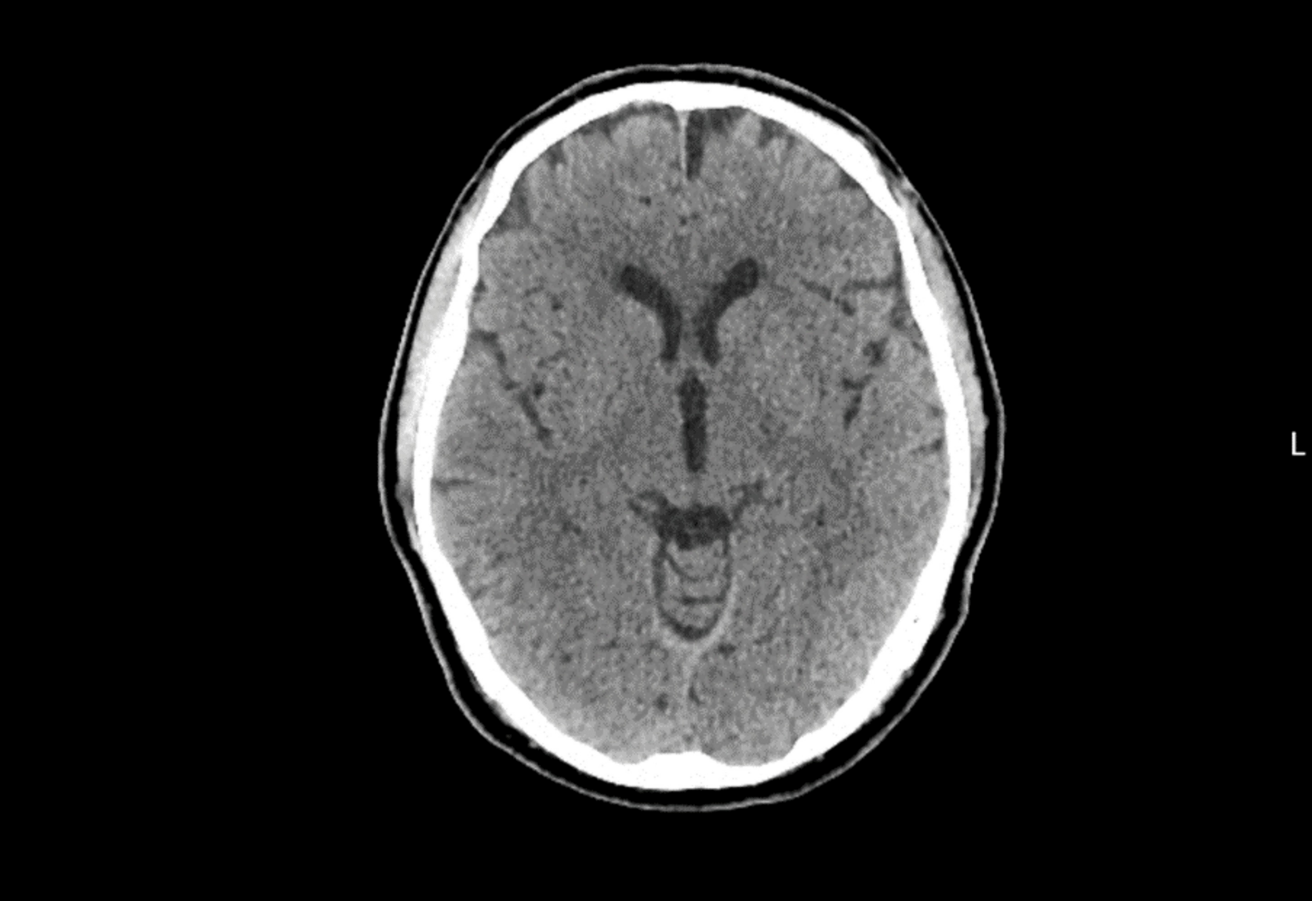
CT scan of the brain showing normal findings
